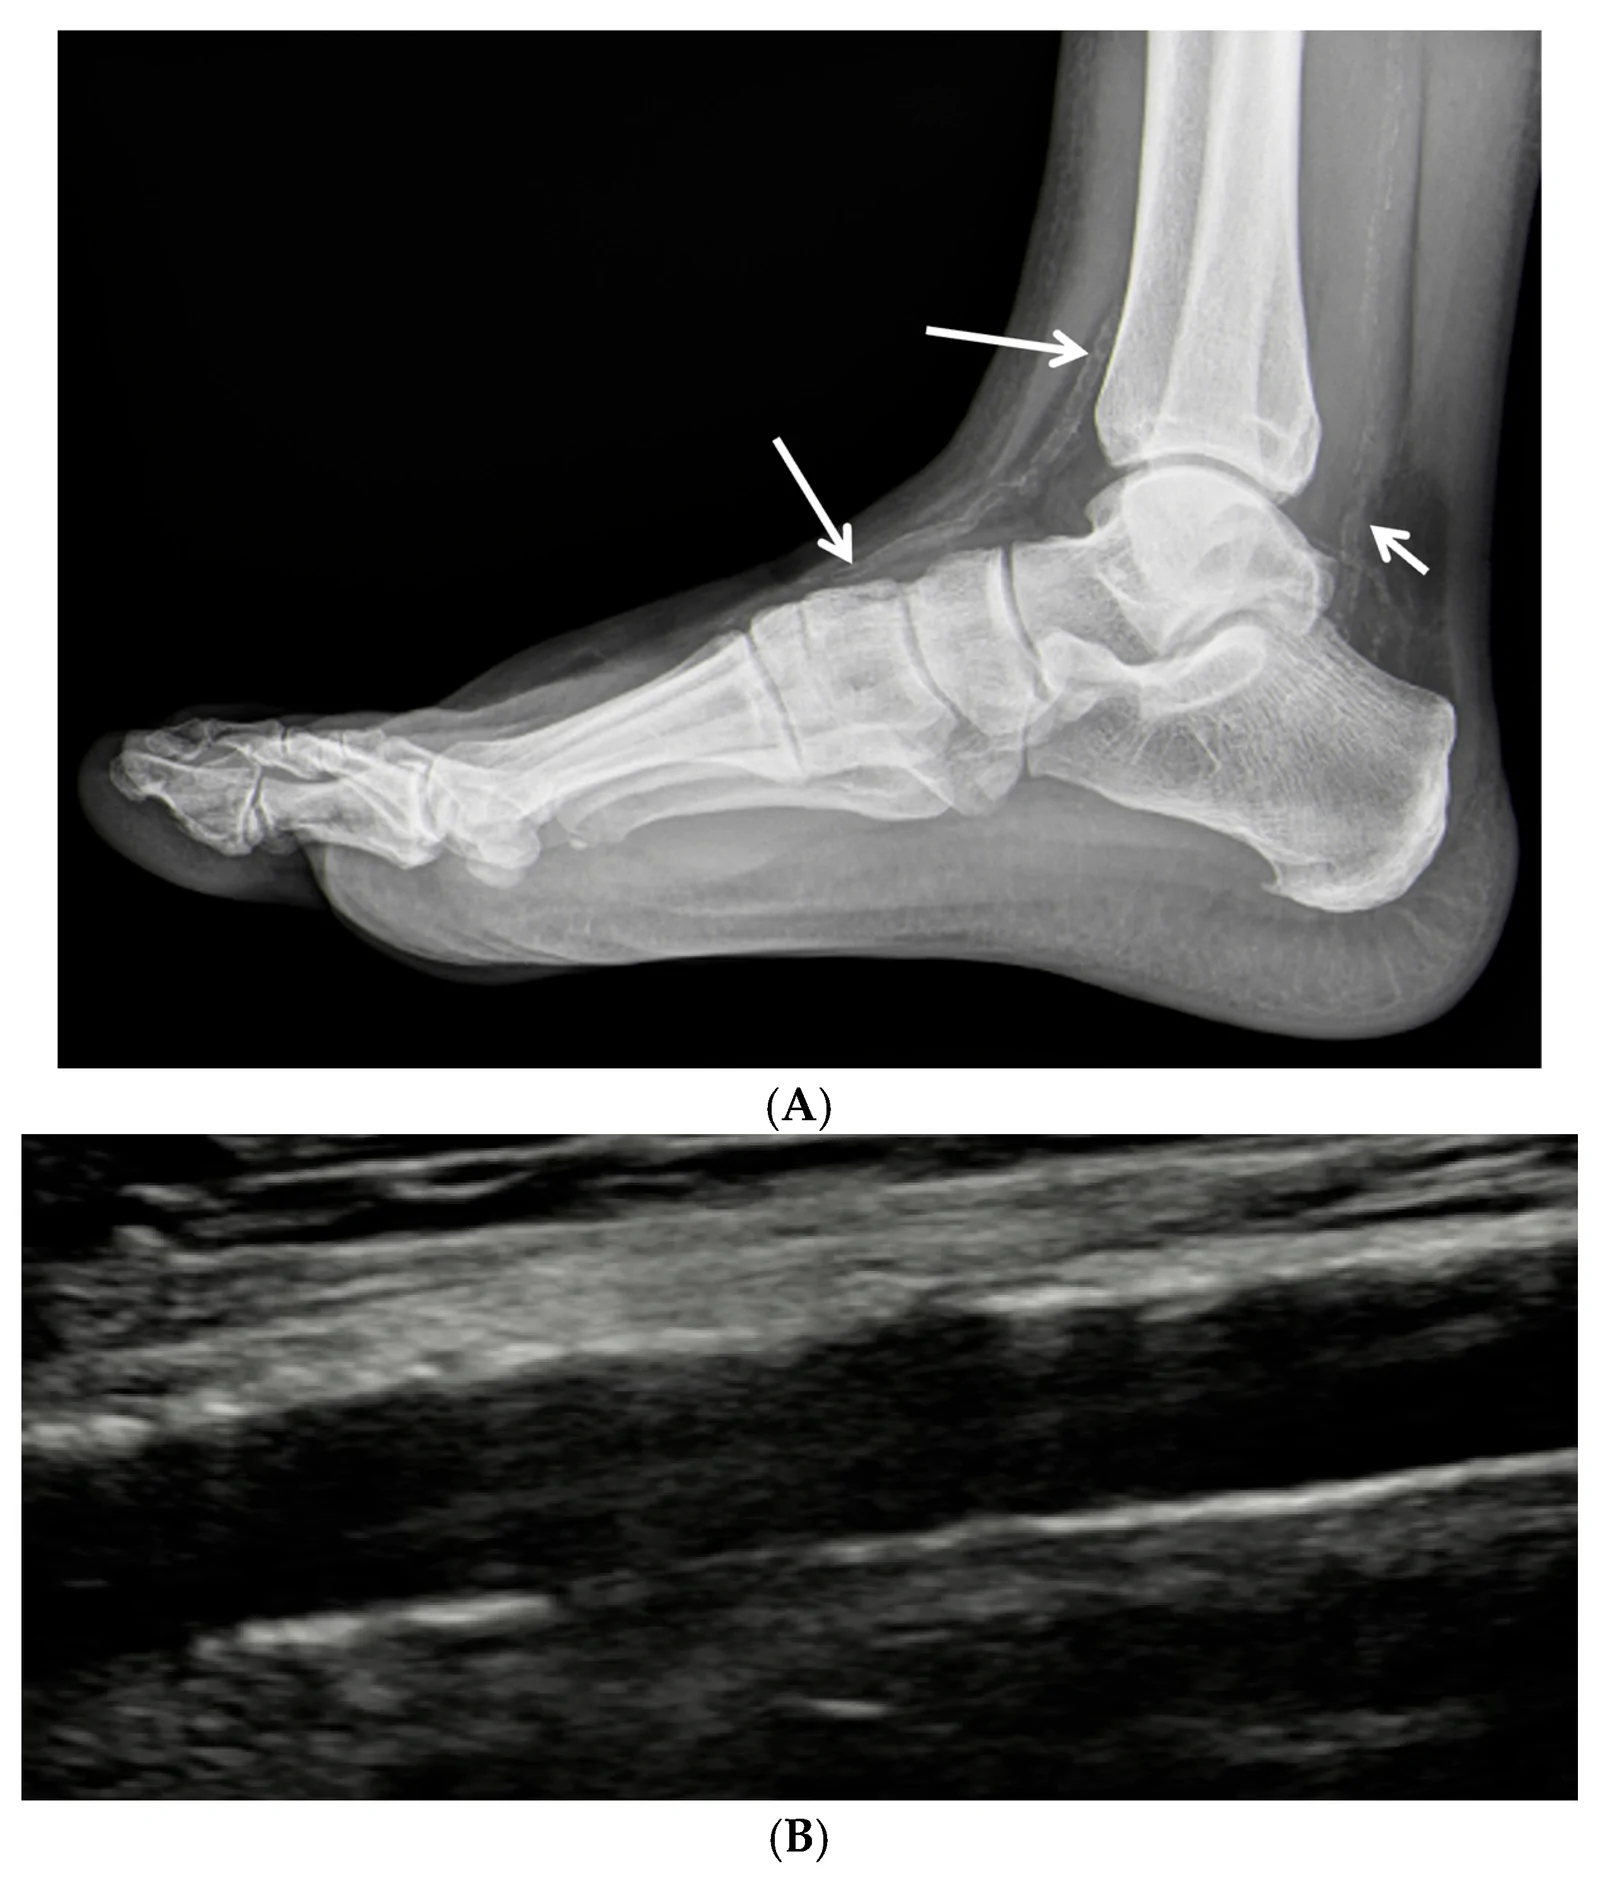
(A) X-ray of the right foot with typical “railroad track pattern” calcifications along the anterior and posterior tibial arteries and dorsalis pedis artery (arrows). (B) B-mode ultrasound of the left superficial femoral artery with medial calcifications: string-of-beads pattern proximally (left side of the image) transitioning to a confluent calcification distally (right side of the image).
